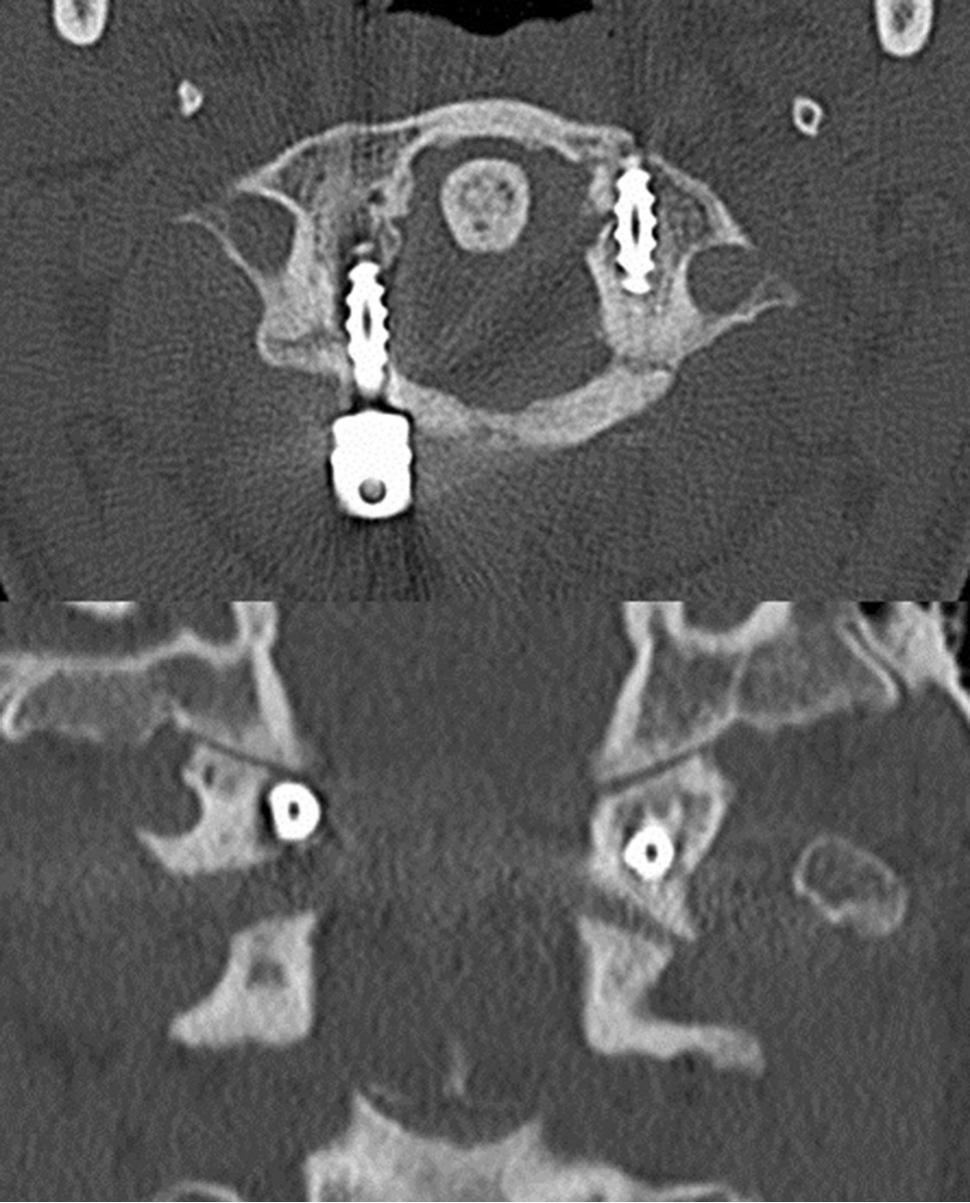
Transversal (*top*) and coronal (*bottom*) CT images of patient 2 after repositioning of the right screw, which is still in a slightly medial but acceptable position. Moderate redislocation of the left lateral mass
